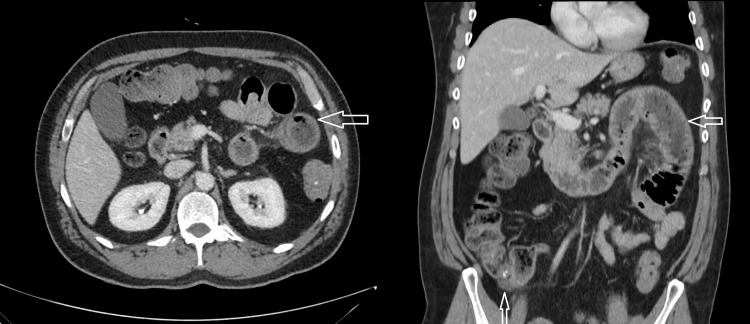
CT Abdomen and Pelvis with IV Contrast. Axial and Coronal view showing short segment of enteritis involving the proximal jejunum (left horizontal arrow). The focal area of fat stranding and small volume of free fluid surrounding the cecum suggests cecum inflammation/caecitis (right lower vertical arrow). Those areas of bowel inflammation may be secondary to the recent diagnosis of vasculitis.
